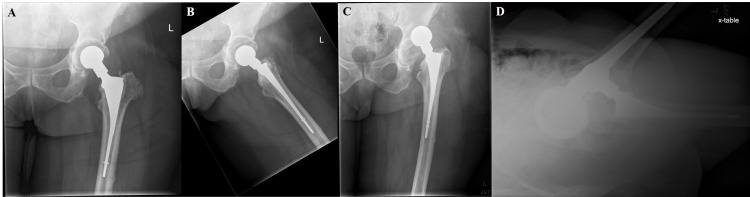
Six-week postoperative anterior-posterior (A) and lateral (B) radiographs after stage 1 of second revision procedure with placement of Zimmer antibiotic spacer. One week later, patient stepped awkwardly and dislocated the spacer (anterior-posterior - C, cross table lateral - D).
